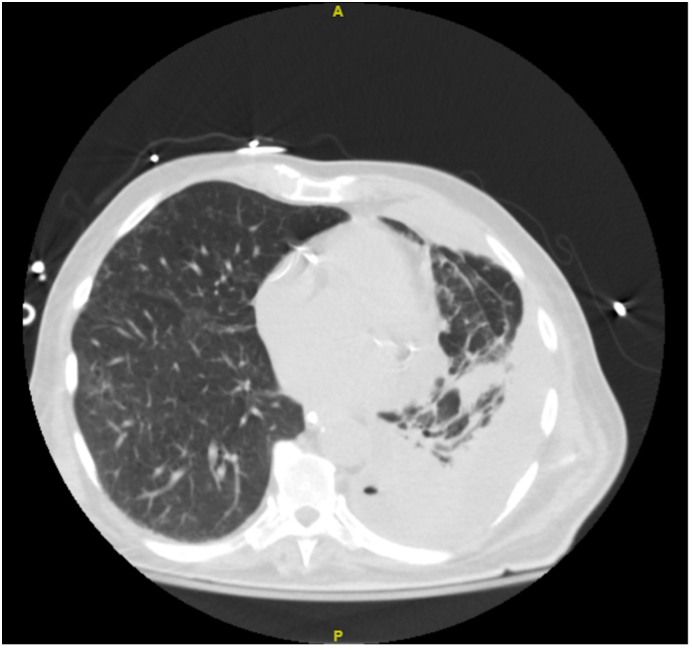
Computed tomography chest showing empyema in the setting of dense left lower lobe air space disease.
